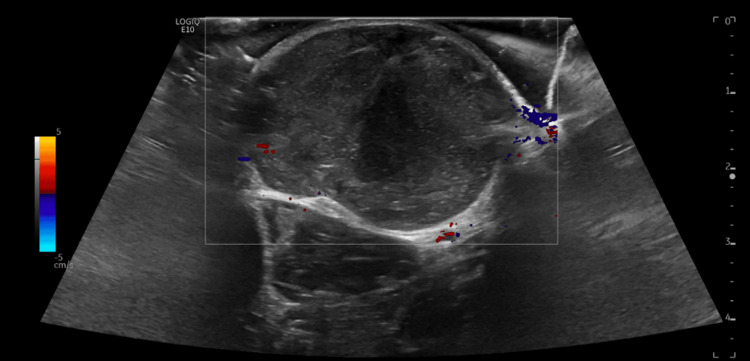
Right-hand dorsal ultrasound demonstrating heterogeneous internal echogenicity
